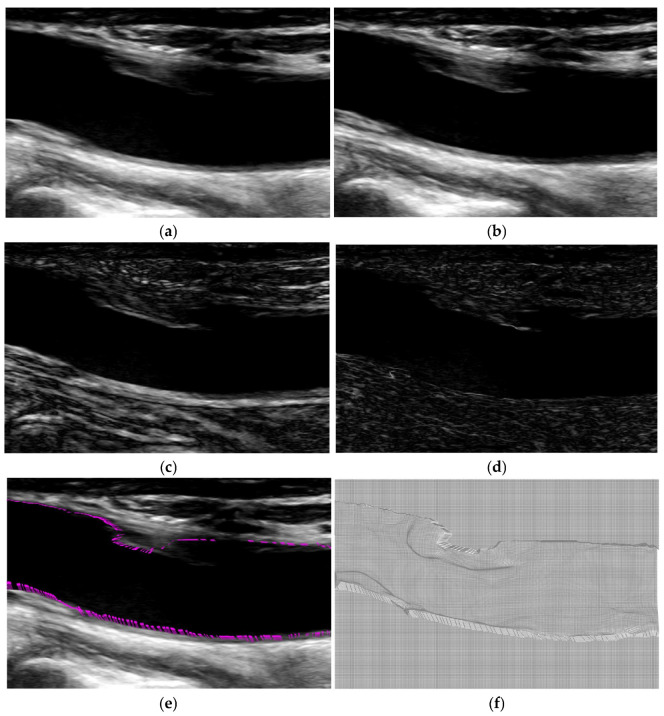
In vivo local carotid artery ultrasound images: (a) the source image, (b) the target image, (c) the difference image between the source and target image, (d) the results obtained by the proposed algorithm, (e) the displacement vector from the boundary in a (red arrows), and (f) the deformation field.
